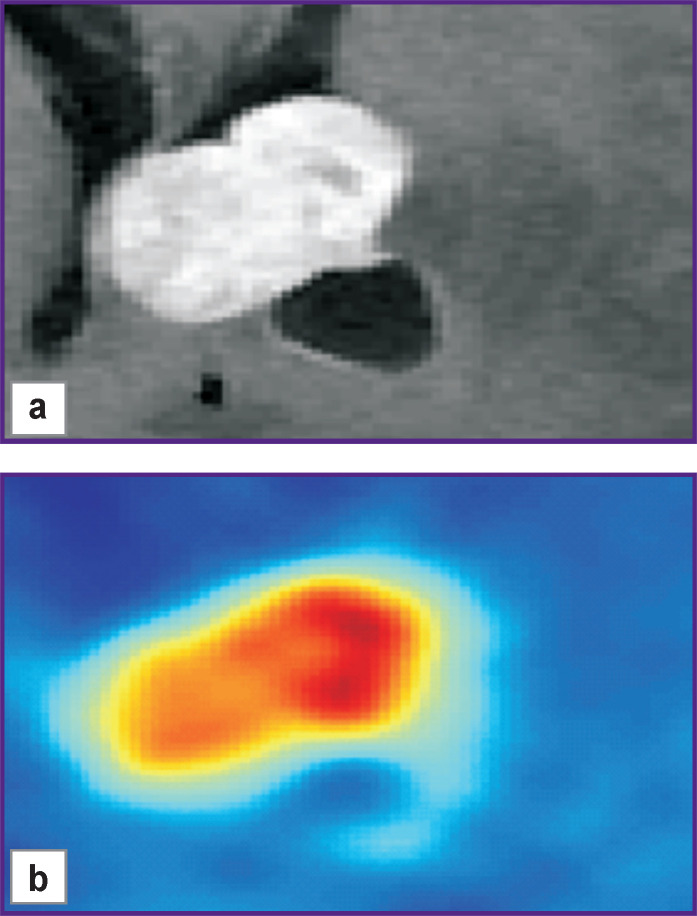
Example of one level in the region of interest set for PET radiomic features calculation in the patient with the diagnosis “glioblastoma localized in the lateral ventricles”: (a) MRI, T1 contrast; (b) ^11^С-methionine PET/CT
